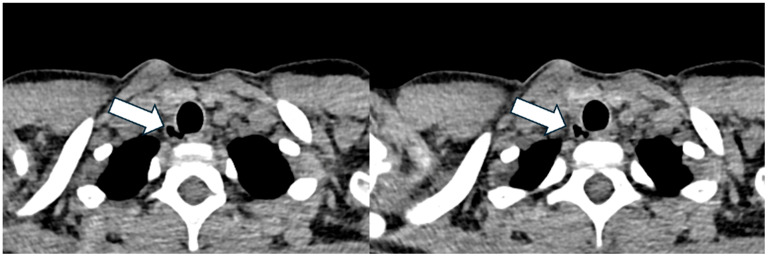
Axial lung window at chest CT showing the tracheal diverticulum at the right posterolateral wall of the trachea in a cystic fibrosis patient (Patient 3). White arrow shows the tracheal diverticulum.
